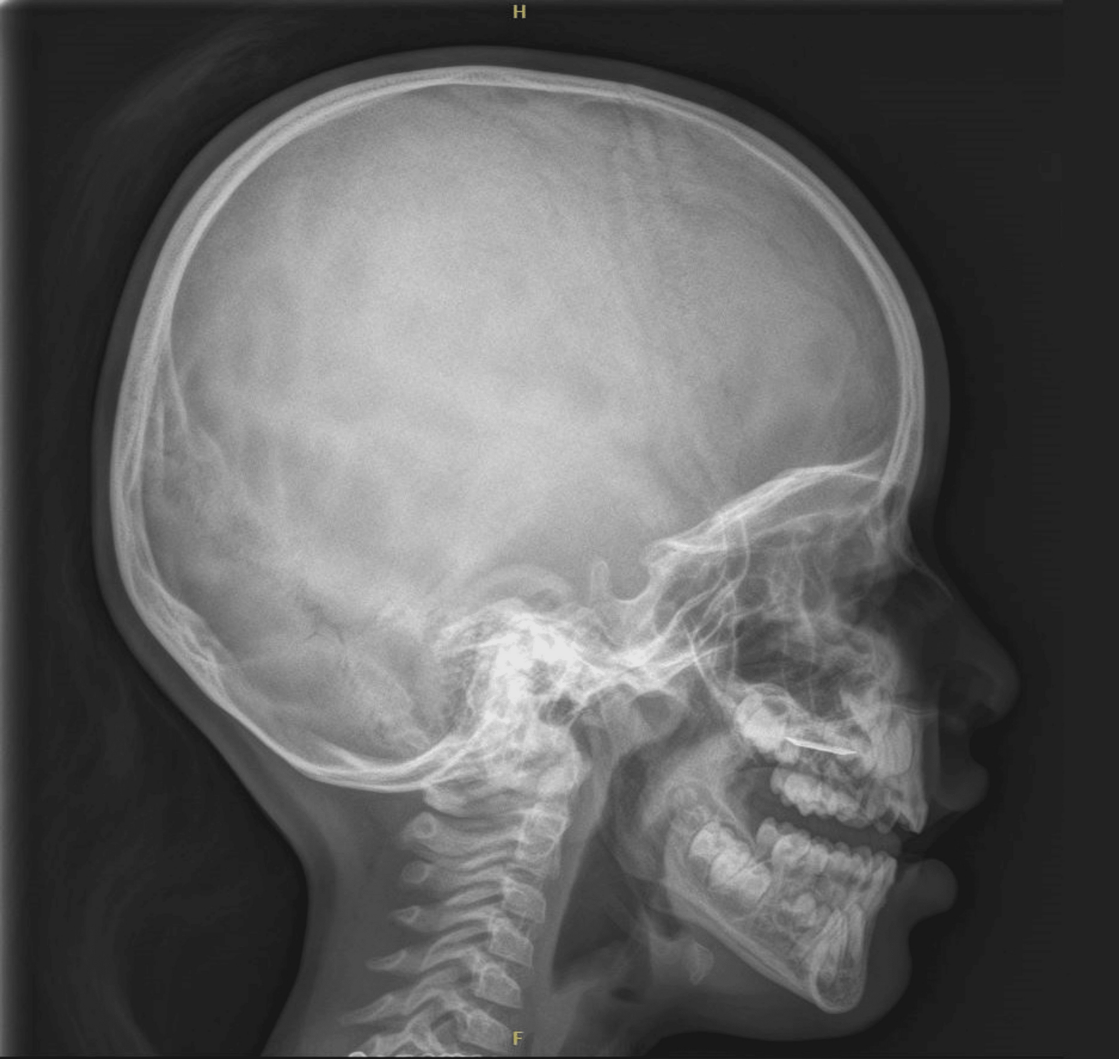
X-ray lateral view showing the foreign body. Lateral view skull X-ray showing a flat and round radiopaque foreign body located at the level of the hard palate.
